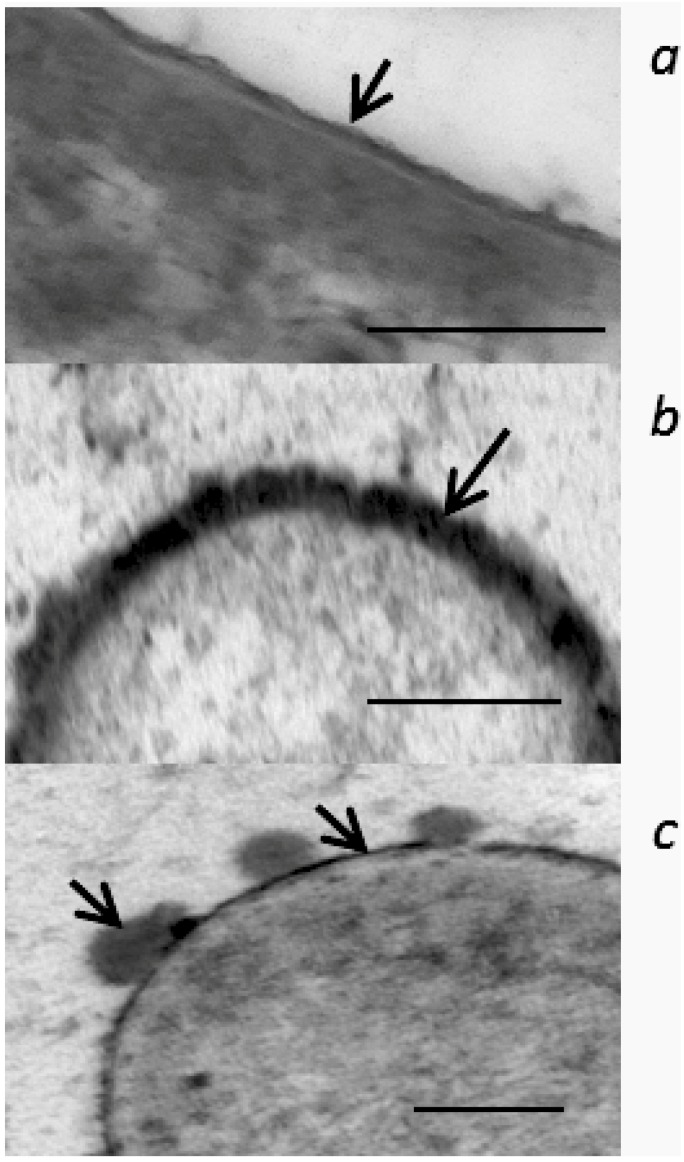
Effect of CT isolated from purple prairie clover (PPC; *Dalea purpurea* Vent.) on OM of *Escherichia coli* (strain ATCC 25922). *E. coli* was aerobically cultured in M9 media containing 0 (*a*) or 50 µg/mL of CT (*b*, *c*) for 12 h. The arrow shows OM of *E. coli* incubated without CT (*a*) and OM that was affected by PPC CT at the concentration of 50 µg/mL (*b*, *c*). Length of bar in each graph indicates 100 nm.
